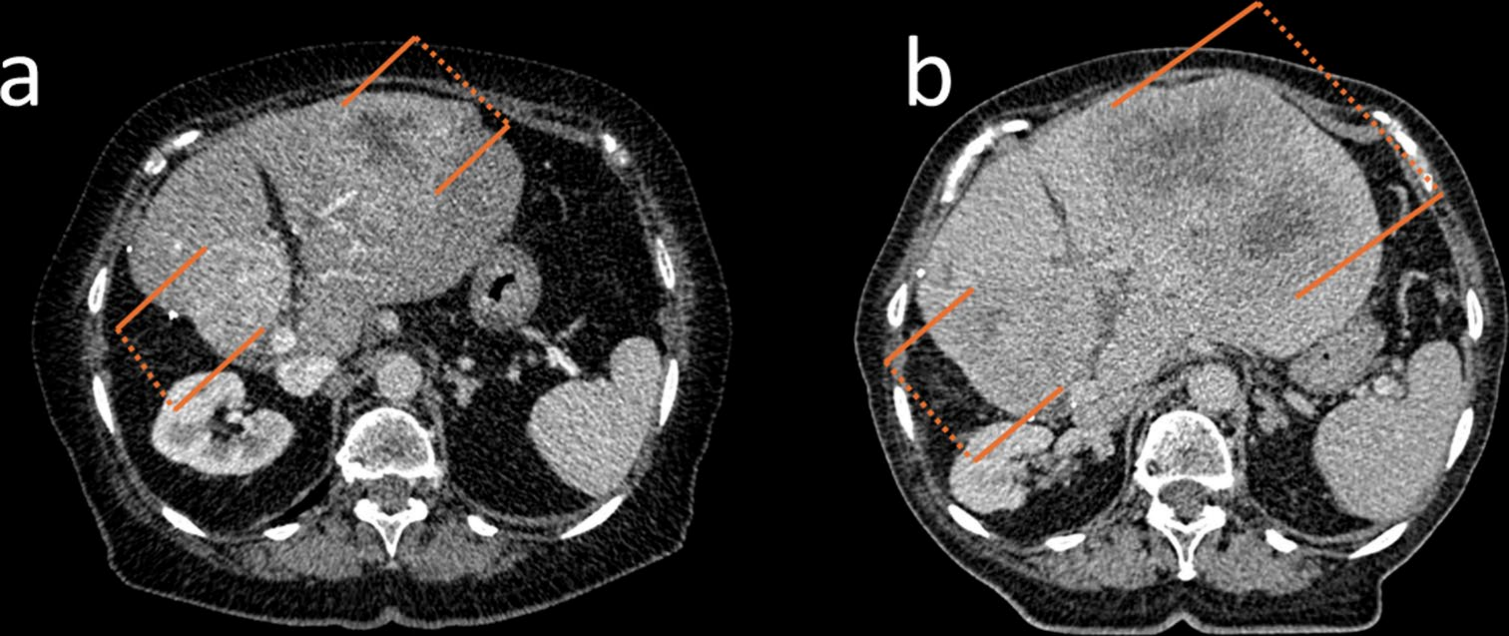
CT-images at baseline (a) and after 4 cycles of PRRT (b) of a representative patient with elevated risk factors at baseline (De Ritis ratio: 1.43, CgA: 12.350 µg/L, and ALP: 120 U/L), who suffered from primary progression and showed an OS of 19 months
